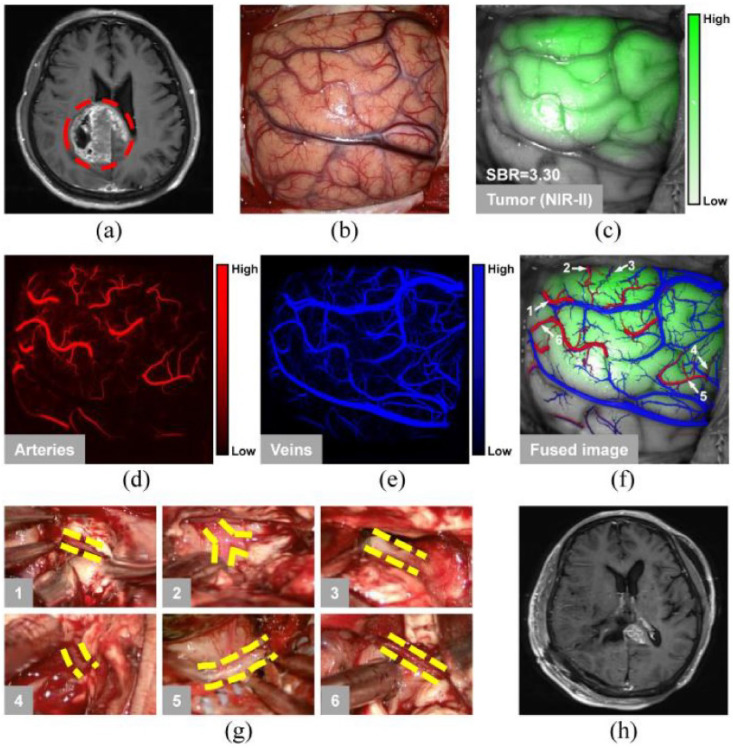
Showcase of blocking feeding arteries and tumor resection of a patient with GBM using the image-guided method. (a) MRI scans before surgery indicated a lesion in the right parietal (red circle). (b) Scan results with visible light after dural opening. (c) Scan of tumor with SWIRF method. NIR-IIb image of the (d) feeding arteries and (e) veins. (f) Integrated image of these separate scans. White arrows 1–6 correspond to the location of the tumor-feeding arteries. (g) Yellow lines show the feeding arteries blocked as a result of tumor resection during surgery. Panel 1–6 correspond to the vessels labeled as 1–6 in (f). (h) MRI scan shows the tumor was resected to the maximum extent after this image-guided surgery. Figure adapted with permission from [55].
